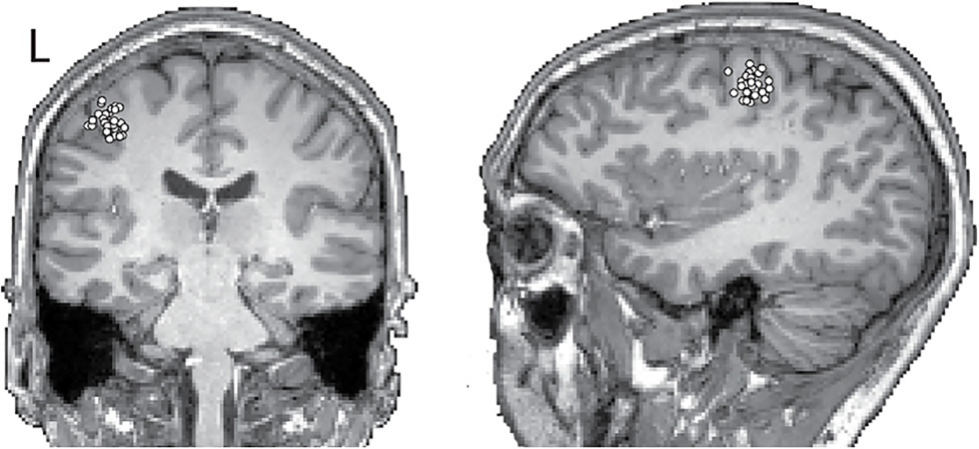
Left hemisphere sources for the 20-Hz activity in one subject. The dots represent 50 equivalent current dipoles, computed for different cycles of 20-Hz oscillations, superimposed on the subject’s magnetic resonance images. The dipoles cluster around the hand knob, which corresponds to the anatomically defined hand motor area.
